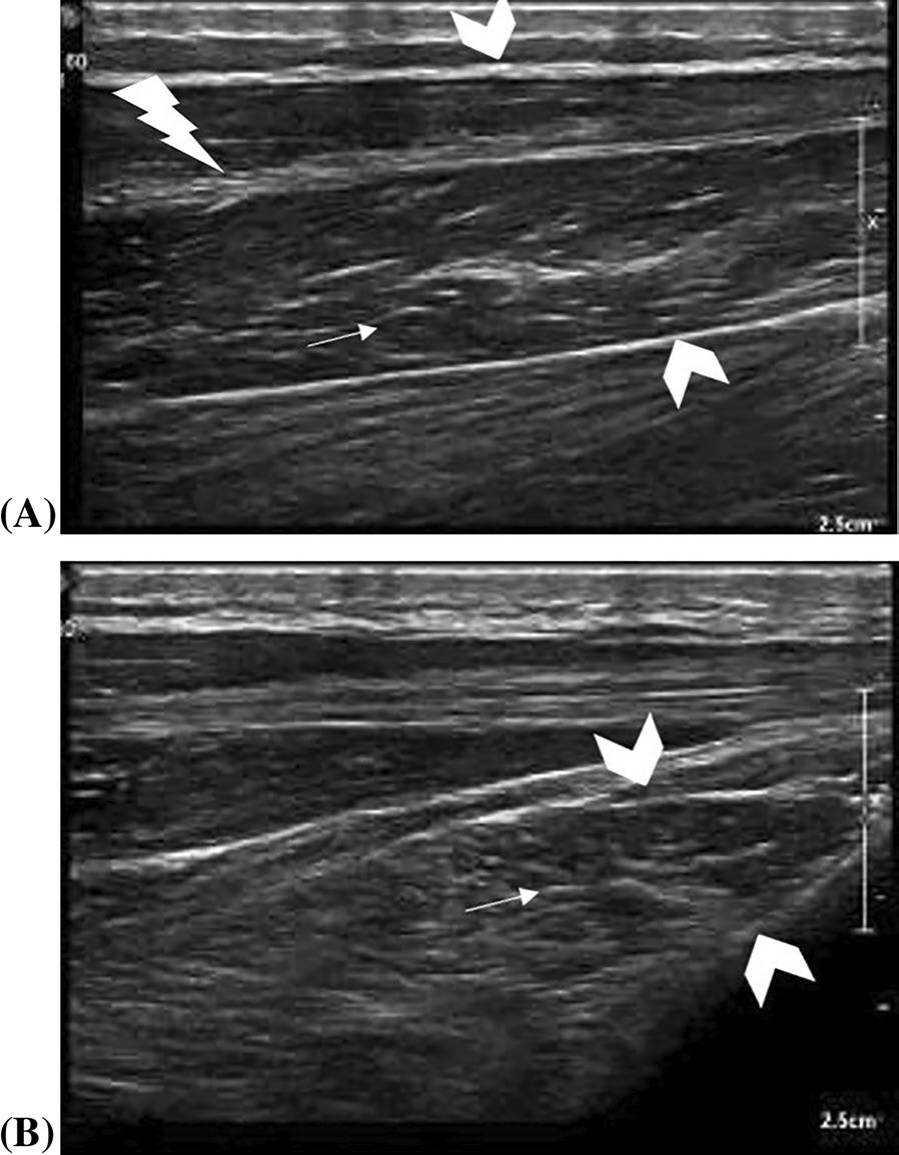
Shows normal skeletal muscle, the soleus, sonographic features. The muscle fibers appear scattered and hypoechoic, enveloped and intertwined by thin echogenic structures of the epi-(thick chevron) and peri-(small arrow) mysium. These arrangements give the muscles a feathery appearance on the longitudinal plane (A) and a mottled appearance on the axial plane (B). Note the central tendon (lightning Bolt) due to the bi-pennate type of soleus muscle
